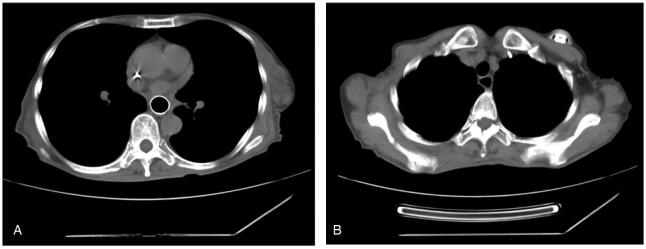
Figure 5. Computed tomography after esophageal stent placement. An esophageal stent (red arrow) was implanted in the lower esophageal stricture (A). Improvement of dilation of the upper esophagus after stent placement (B).
